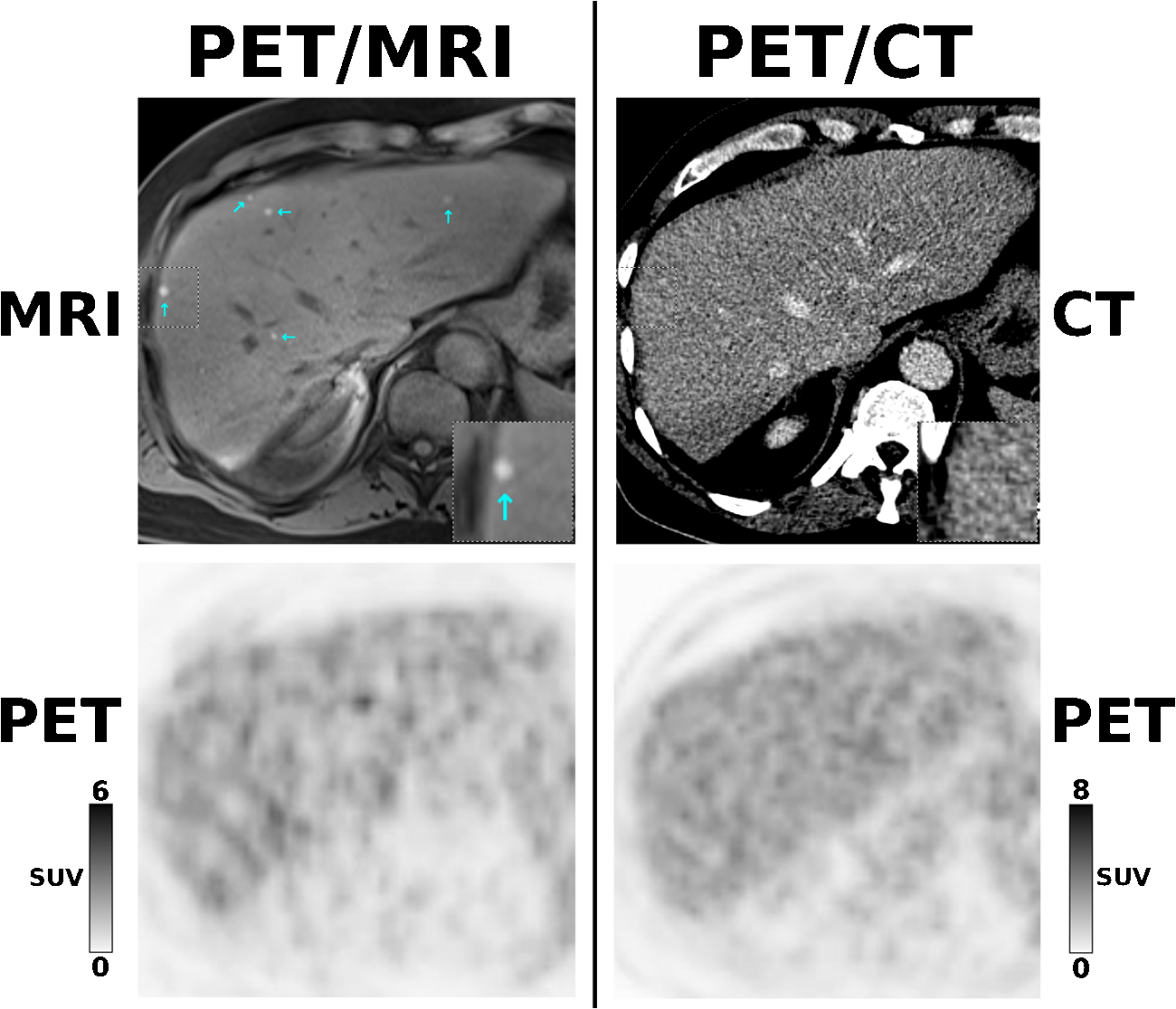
A 59-year-old patient with malignant melanoma stage IV, referred for follow-up after ipilimumab treatment. While [^18^F]FDG-PET is unremarkable for both PET/MRI and PET/CT, the contrast-enhanced MRI component of PET/MRI depicts multiple, newly developed small liver metastases (cyan arrows) that are not visualized by the contrast-enhanced CT component of PET/CT (× 2 magnifications of the segment VIII lesion in right lower corners). This changed the diagnosis to progressive disease and led to a switch from ipilimumab to PD1 antibody treatment
